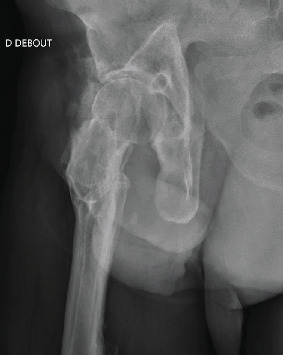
Lateral view of the X-ray in Figure 5.
